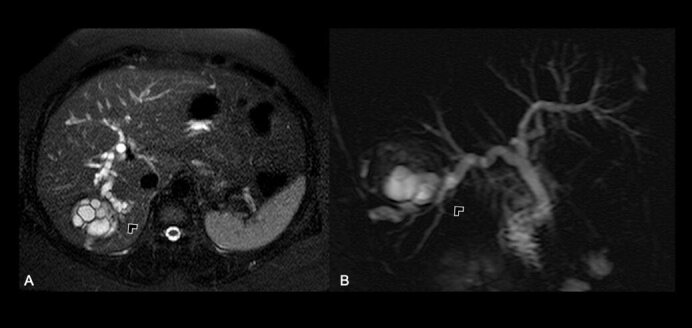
Biliary tree communication. (A) Axial fat-saturated T2-weighted image; (B) Heavily T2-weighted image sequence.
